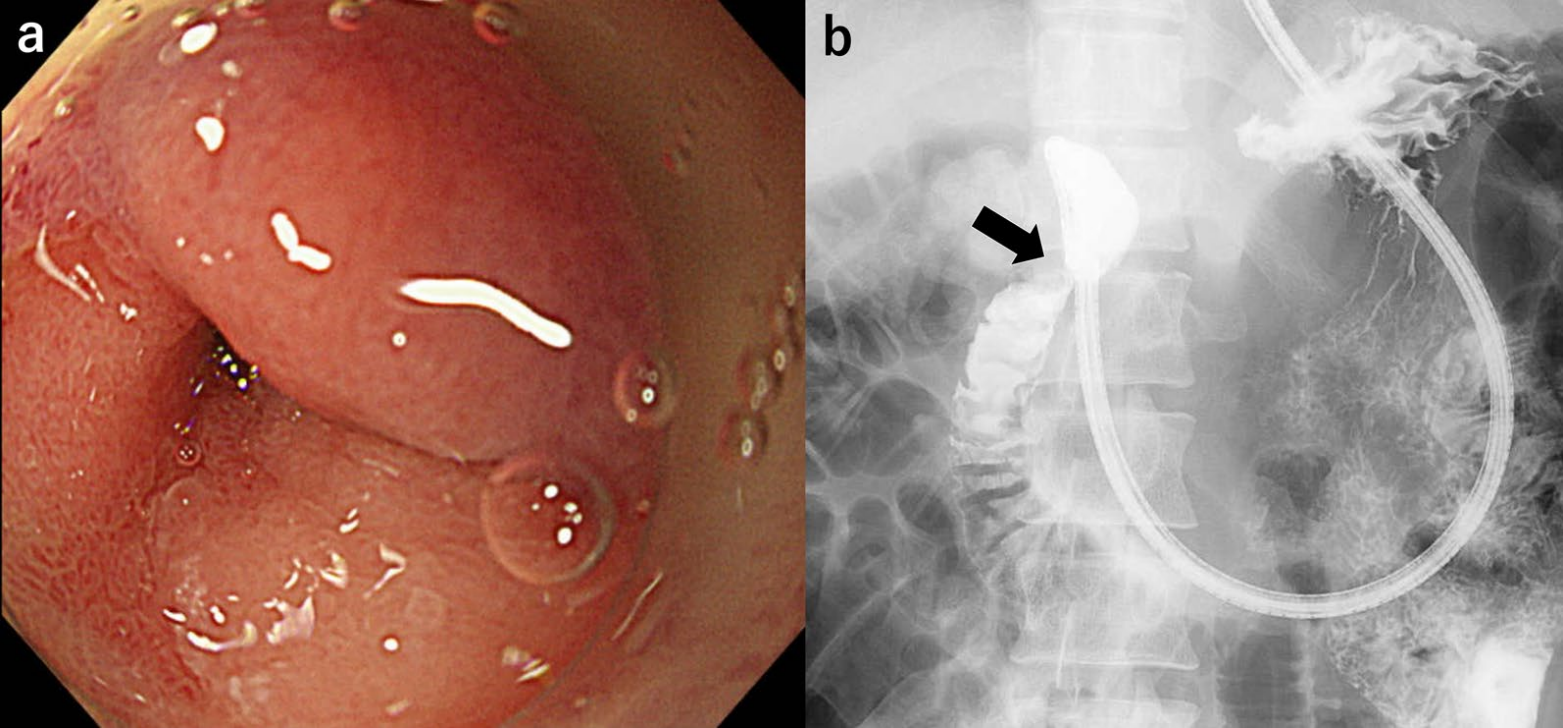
Images of Gastrointestinal (GI) Endoscopy and Upper GI Radiography. a Endoscopy showing a duodenal pinhole stricture. The mucosa exhibited edema and redness; however, no clear ulcerations were observed. b Upper GI contrast showing a short stricture at D1/D2 of the duodenum (arrow)
